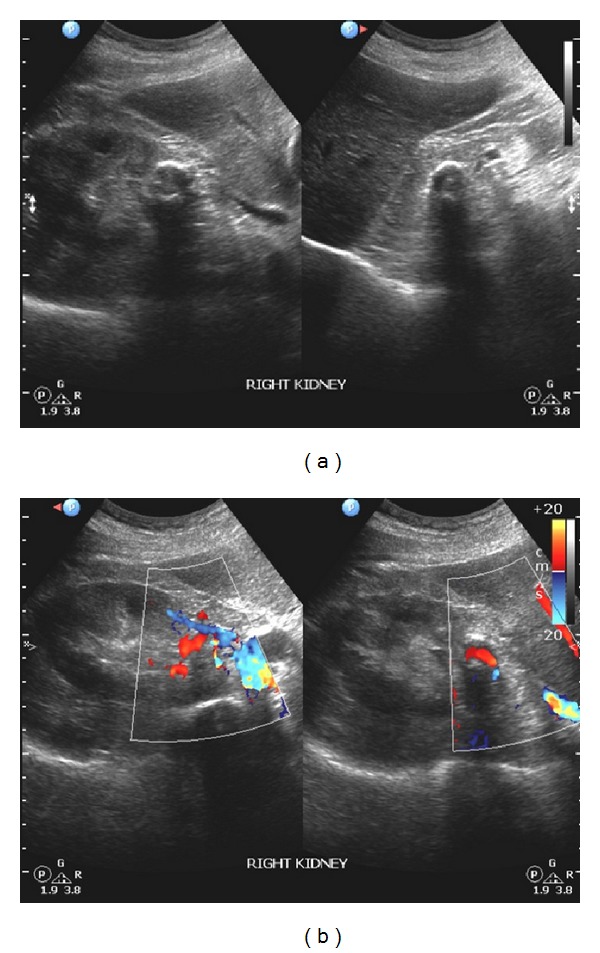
(a) This grey-scale transabdominal ultrasonography image of the right renal area was acquired with a curvilinear multifrequency transducer. It incidentally revealed a curvilinear reflective line with acoustic shadow behind it. This structure was located near the renal pelvis. (b) Color Doppler imaging demonstrated blood flow within the previously described structure. Thus, the structure should be vascular in its nature.
